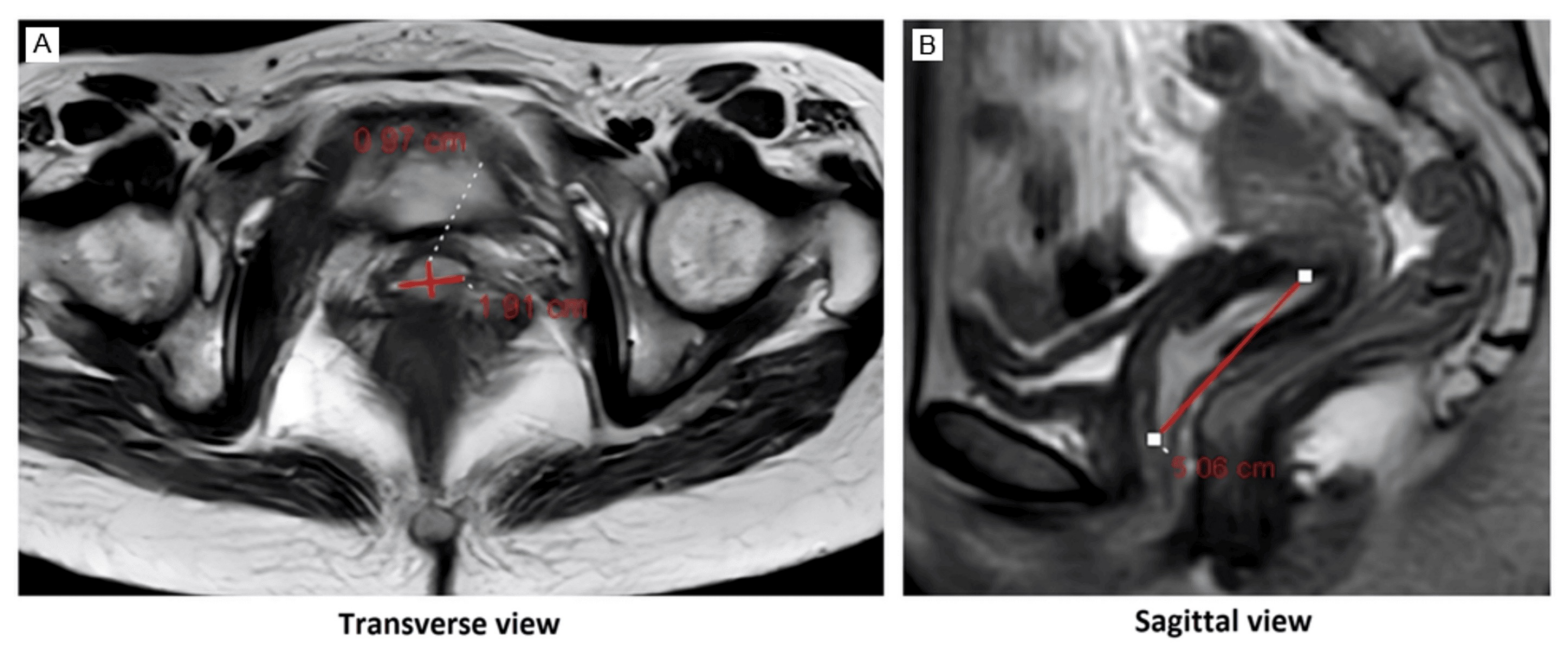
(A, B) MRI scan with contrast showing a large cervical pedunculated cervicovaginal polyp arising from the anterior cervical wall seen widening the cervical canal and extruding from the external orifice to the proximal upper vagina The polyp measures about 5.01 × 1.91 × 0.97 cm in CC, TS, and AP diameters, respectively MRI: magnetic resonance imaging; CC: craniocaudal; TS: transverse; AP: anteroposterior
